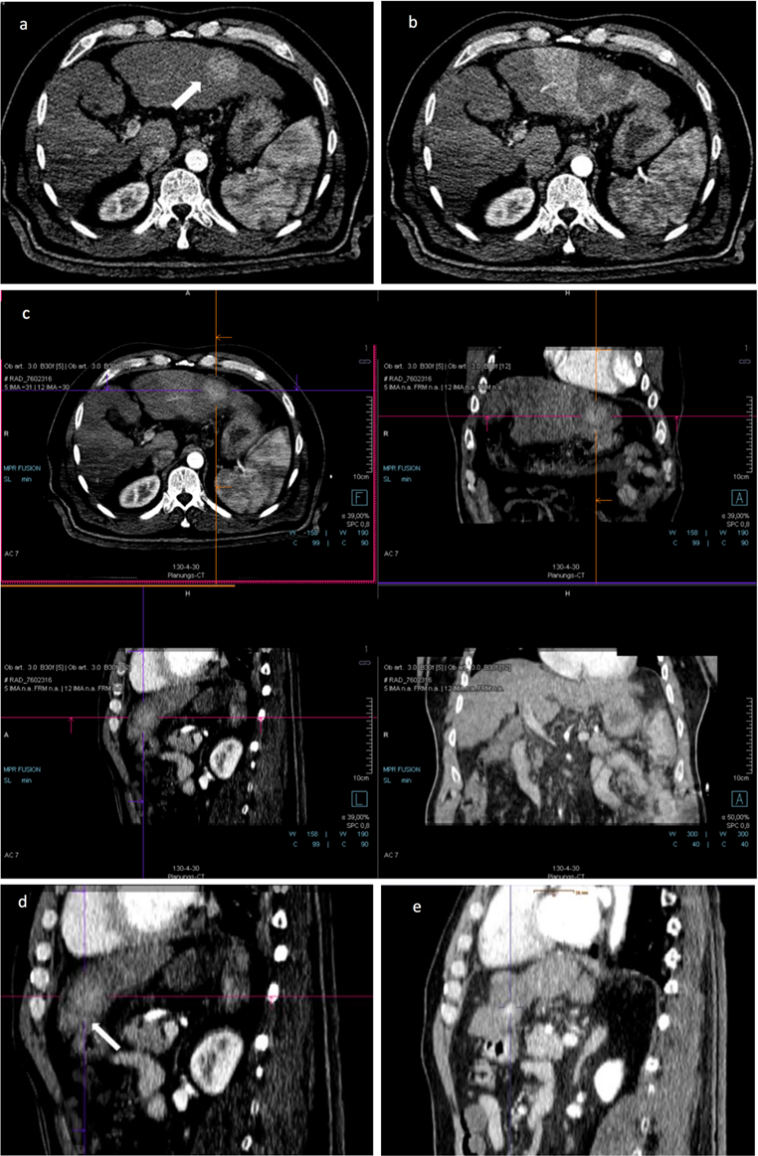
A 63-year-old male patient treated with stereotactic RFA. a The arterial phase of pre-SRFA CT scan depicting a single HCC lesion (arrow) with a maximal diameter of 3.4 cm in liver segment II. b The arterial phase of post-SRFA CT scan with transient hyperemic rim around the ablation zone. c The after fusion of pre- and post-SRFA CT scan with manual registration by slight shifting (translation and rotation) in axial, coronal, and sagittal planes referring to intrahepatic structures such as vessel bifurcations. d The sagittal plane of CT image fusion with a MAM of 2.2 mm in clock position 5–6 h (arrow). e Local tumor progression after stereotactic RFA: contrast-enhanced CT scan 20.2 months after stereotactic RFA revealing a hypervascular, contrast-enhancing lesion in the arterial phase and with washout in delayed phase that is located immediately adjacent to the ablation zone
